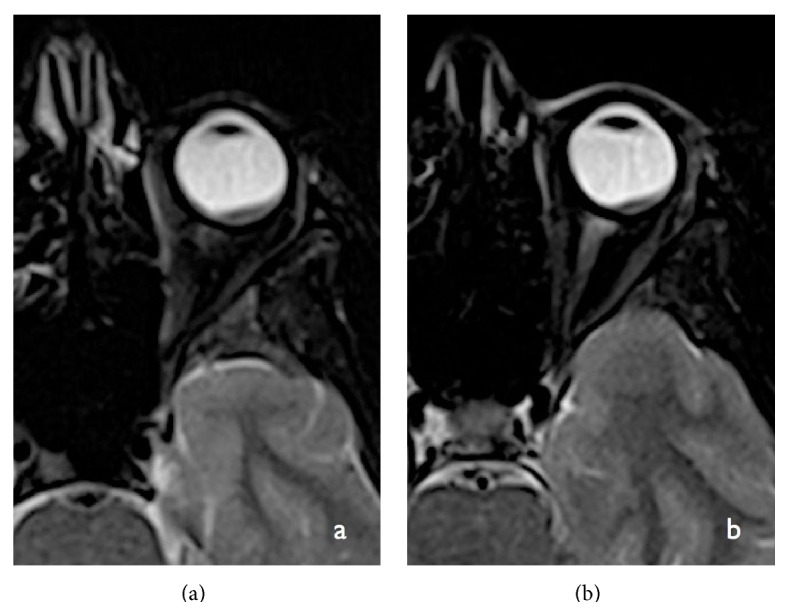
Axial T2-FLAIR MRI image showing that the lesion appears hypointense to gray matter with typical signal intensities of the optic nerve.
